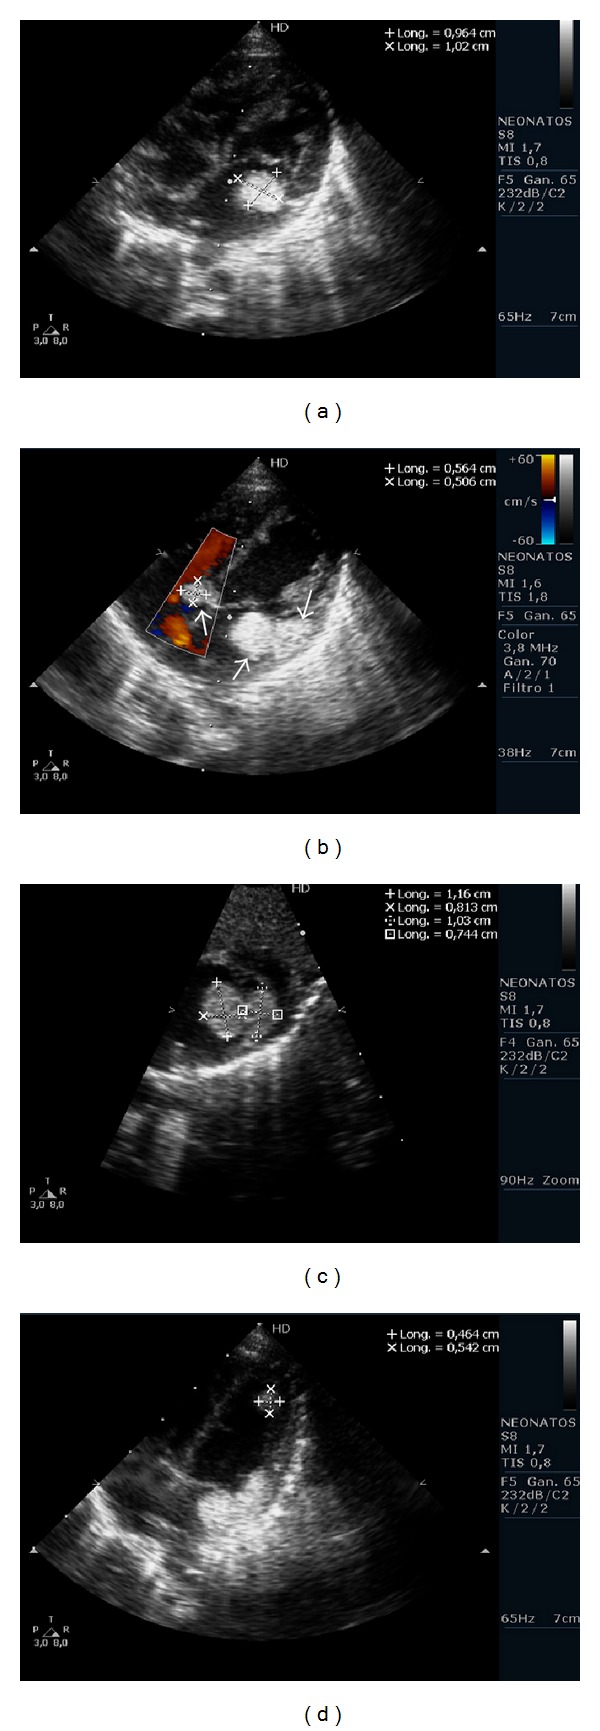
Views of different sizes and localisations of rhabdomyomas at the time of diagnosis. (a) Four-chamber view with intracavitary masses in mitral valve of 0,9 × 1 cm. (b) Four-chamber doppler-colour view of intracavitary mass of 0,5 × 0,5 cm in tricuspid valve, one intracavitary mass in mitral valve and one intraparietal mass in lateral wall of left atrium (arrows). (c) Subcostal view of left ventricule. One intracavitary mass and one intraparietal mass in lateral face of left ventricule. (d) Four-chamber view of intraparietal tumour in left ventricule apex.
